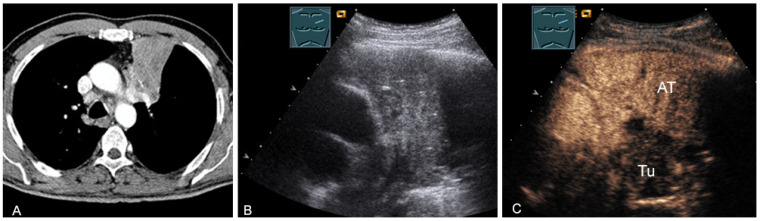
A patient with mediastinal mass on CT (provided by Prof. Dr. Andreas H. Mahnken, Marburg, Germany) (A), B-mode US (B), and CEUS (C), differentiating bronchial carcinoma (TU; hypoenhancing) from downstream atelectasis (AT, marked enhancement).
